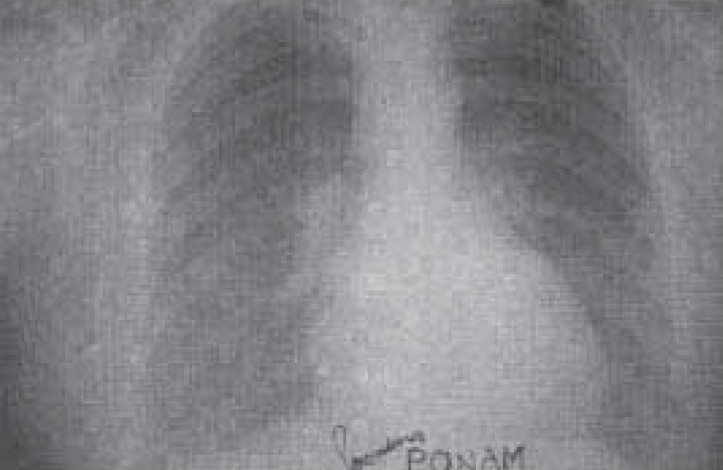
Showing Chest X-ray PA view of patient.
